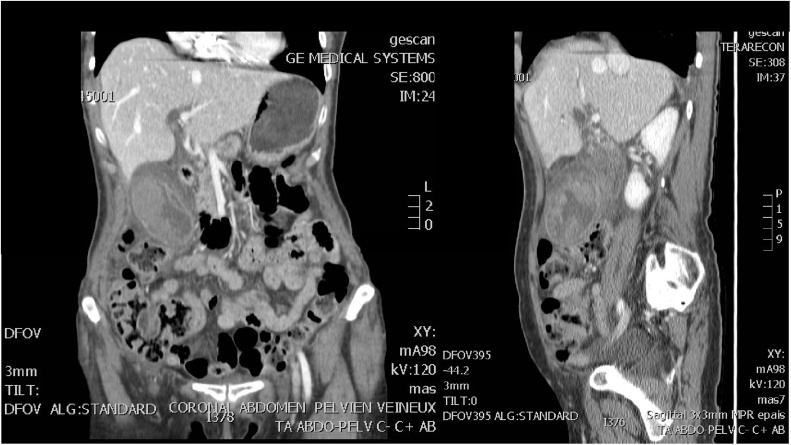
Coronal and sagittal enhanced CT images showing a markedly distended and wall-thickened gallbladder.
